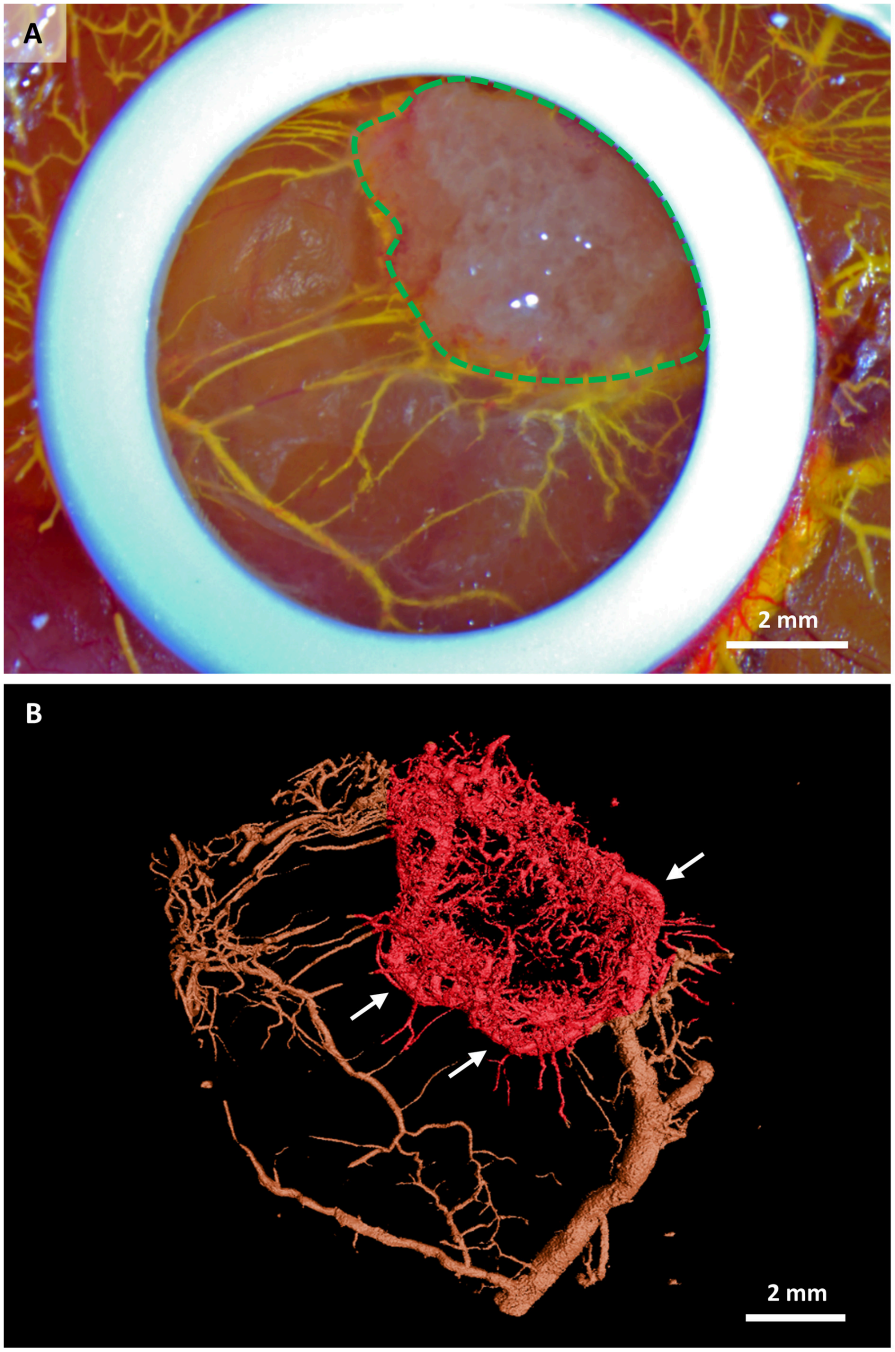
Stem cell-seeded scaffolds perfused with MicroFil® after their *in ovo* culture. (A) Macroscopic view of a scaffold (green dashed line) immediately after perfusion with yellow MicroFil®. The silicone ring is seen in white, vasculature in yellow. (B) Microcomputed tomography image of the vascular cast within the scaffold (red color). Vasculature outside the scaffold area is marked in brown. New capillaries are sprouting from one large vessel that encircles the scaffold (white arrows).
